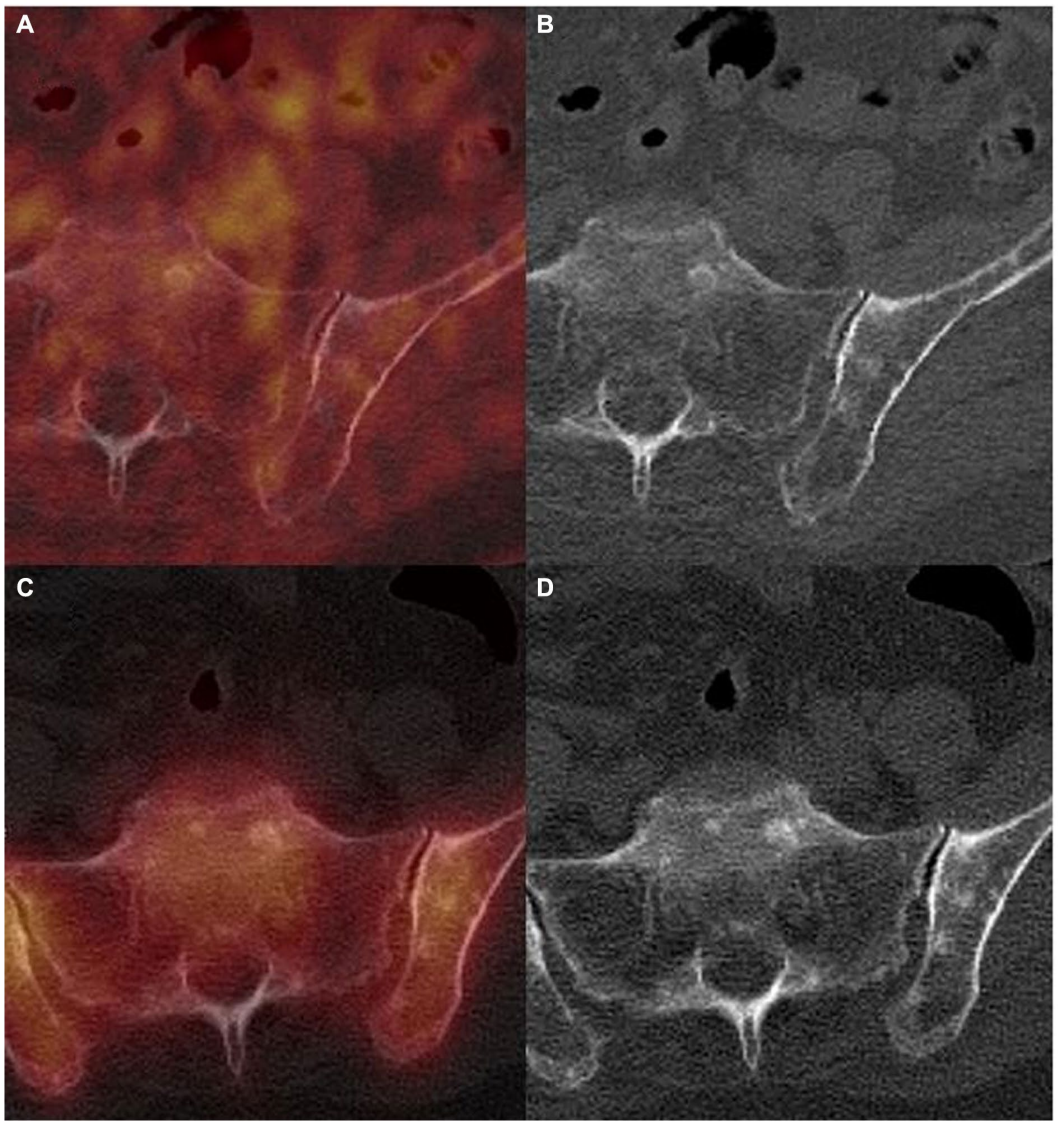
^18^F-FDG PET/CT (A,B) and ^99m^Tc-HMDP SPECT/CT (C,D) in a 75 years-old woman referred for initial staging of an invasive lobular carcinoma of the breast, showing multiples subcentimetric sclerotic bone lesions, linked to a diffuse bone invasion on the rest of the scans (not shown), without ^18^F-FDG and ^99m^Tc-HMDP uptake, corresponding to bone metastases confirmed by biopsy.
